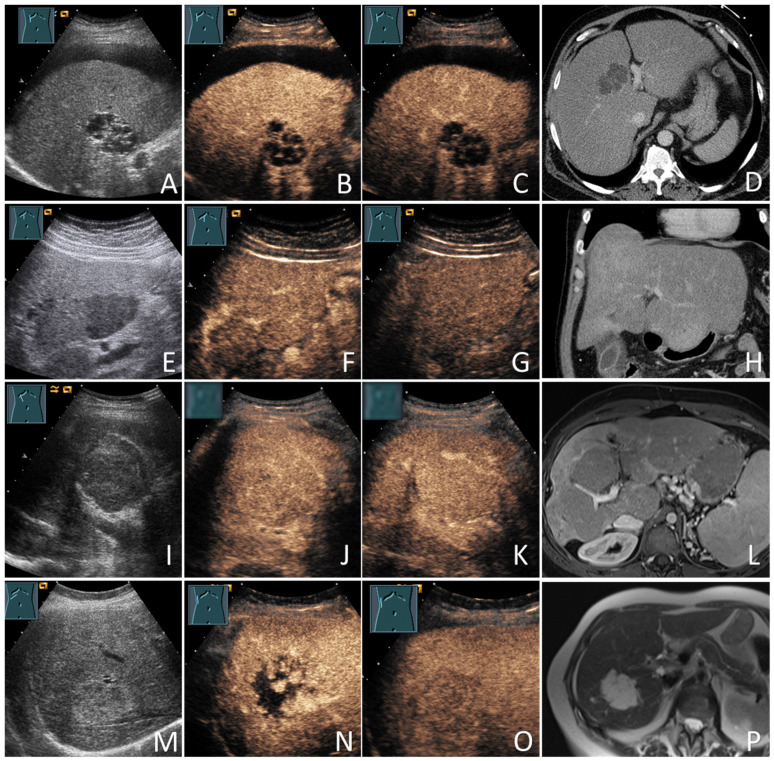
Benign focal liver lesions (bFLLs) in patients with liver cirrhosis (LC). Liver cyst (A–D): hypoechoic FLL with multiple septa on grey-scale ultrasound (A); hypoechoic FLL with arterial phase isoenhancement of the septa (B); hypoechoic FLL without washout in the delayed venous phase (C); the corresponding CT of the FLL (courtesy of Prof. Dr. Andreas H. Mahnken, Department of Radiology, University Hospital Marburg) (D). Focal fat sparing (E–H): hypoechoic FLL on grey-scale ultrasound (E); hypoechoic FLL with isoenhancement on the arterial phase (F); hypoechoic FLL with isoenhancement on the delayed venous phase (G); the corresponding CT of the FLL (courtesy of Prof. Dr. Andreas H. Mahnken, Department of Radiology, University Hospital Marburg) (H). Regenerative nodules (I–L): large central isoechoic lesion on grey-scale ultrasound (I); large central isoechoic lesion with isoenhancement on the arterial phase (J); large central isoechoic lesion with isoenhancement on the delayed venous phase (K); the corresponding MRI of the FLL (courtesy of Prof. Dr. Andreas H. Mahnken, Department of Radiology, University Hospital Marburg) (L). Hemangiomas (M–P): large central echogenic lesion on grey-scale ultrasound (M); large central echogenic lesion with nodular enhancement on the arterial phase (N); large central echogenic lesion with isoenhancement on the venous phase (O); the corresponding MRI of the FLL (courtesy of Prof. Dr. Andreas H. Mahnken, Department of Radiology, University Hospital Marburg) (P). The lesions remained stable over a follow-up period of over 92 months.
